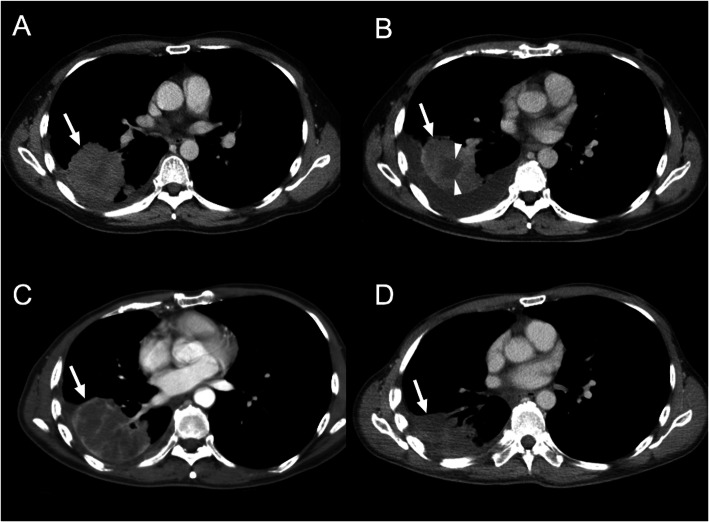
Tumor necrosis indicates early good response in non-small cell lung cancer (NSCLC) receiving targeted therapy. A 53-year-old man diagnosed with NSCLC (positive EGFR exon 20 insertion mutations) and received afatinib therapy. A Pretreatment contrast-enhanced (CE) axial CT scan shows an enhancing tumor (arrow), with diameter of 64 mm. B, C Pseudo-progression with increased tumor size (arrow) was observed in 3 months (B) (67 mm in tumor diameter) and 8 months (C) (74 mm in tumor diameter) after targeted therapy. Simultaneously, the progression of focal tumor necrosis (arrowheads in figure B) to diffuse tumor necrosis (C) was also observed. D Shrinkage of tumor mass (50 mm in diameter) was observed 15 months after therapy
